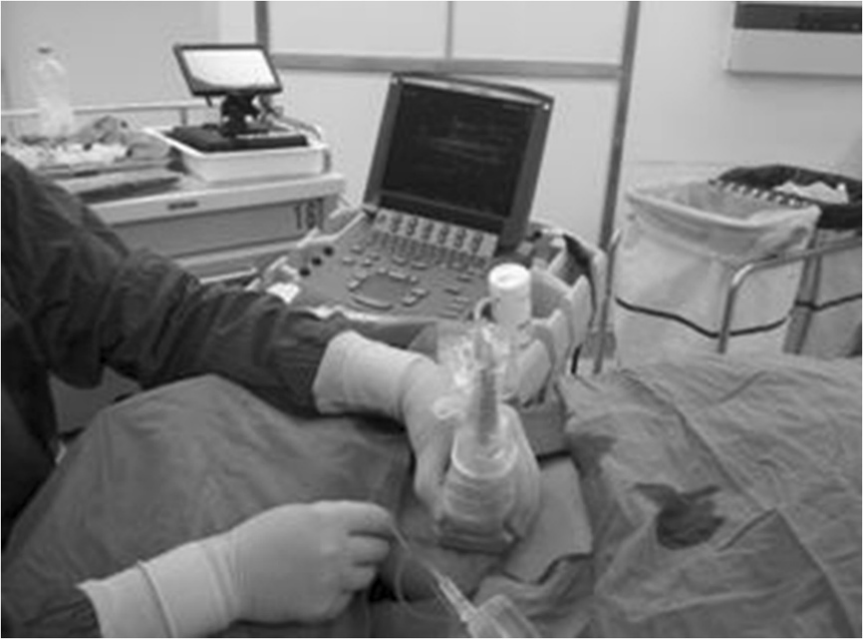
Position of the ultrasound probe relative to the needle (needle-in-plane technique) for the ultrasound-guided anterior cervical cutaneous nerves block (CCNB).
